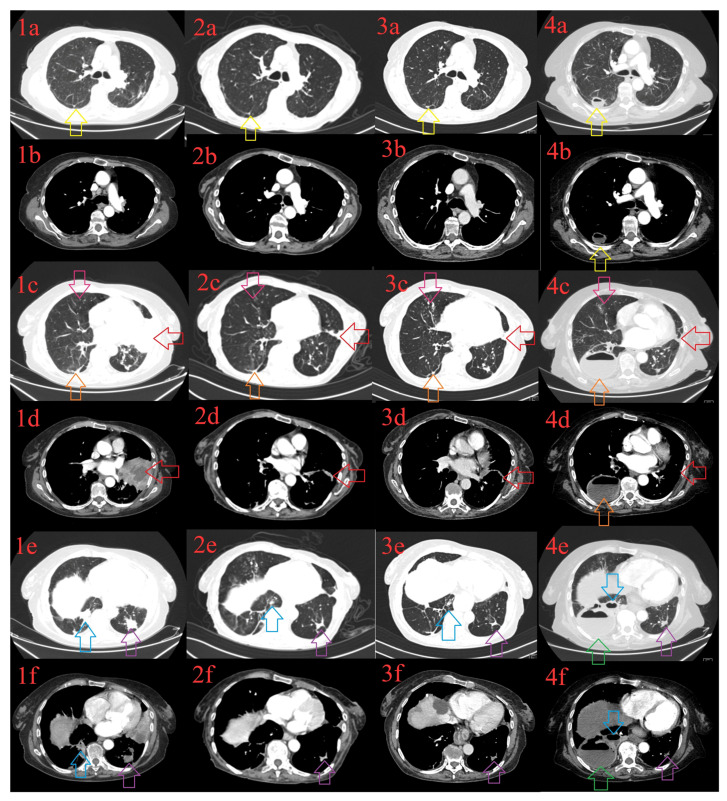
Figure 2 shows four consecutive chest CT examinations, with mutual time distances of 6, 3, and 6 months, throughout the disease course in the three sections of the chest in lung window (Images 1a,c,e–4a,c,e) and soft tissue window (Images 1b,d,f–4b,d,f), depicting the temporal versatility of caseous granulomatous lesions as a hallmark of GPA. At the level of bifurcation of trachea, in the posterior segment of the upper right lobe, there was a thin subpleural band with a solid nodule depicting mild size progression throughout three consecutive CT examinations (Images 1a–3a, yellow arrow) that, after 6 months, became a caseous granuloma with excavation (4a,4b, yellow arrow). At the level of the left atrium, in the lingula of the upper left lobe, there was caseous consolidation (1c,1d, red arrow), previously misunderstood as an excavating carcinoma, that became a thick subpleural band in the following three CT examinations (2d–4d, red arrow). At the same level, in the apicobasal segment of the lower right lobe, there was a thick subpleural band with a solid nodule in three consecutive CT examinations (1c–3c, orange arrow) that, after 6 months, became a caseous granuloma with excavation (4c,4d, orange arrow). Also, there was development of centrilobular nodules in a “tree-in-bud” pattern in the middle lobe throughout four consecutive chest CT examinations (1c–4c, pink arrow). At the level of the left ventricle, in the lower right lobe in medio-basal segment, there was caseous granuloma in resolution (1e,1f, blue arrow) that became a thick subpleural band in the two following CT examinations (2e,3e, blue arrow) but evolved to a larger caseous granuloma after six months (4e,4f, blue arrow), with development of similar lesions in the neighboring posterobasal segment (4e,4f, green arrow). At the same level, in the laterobasal segment of the lower left lobe, there was a caseous granuloma (1e,1f, purple arrow), which became a thick subpleural band in the following three CT examinations (Images 2e,f–4e,f, purple arrow). Additional lung CT images are provided in Figures S2 and S3 (Supplementary Materials).
